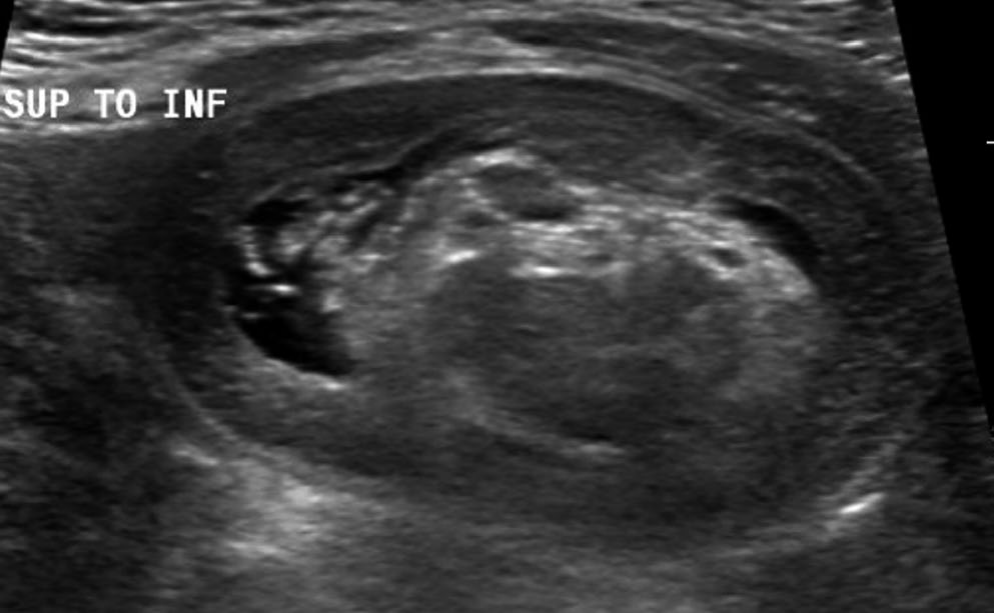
Abdominal ultrasonography shows target sign due to large ileocolic intussusception
